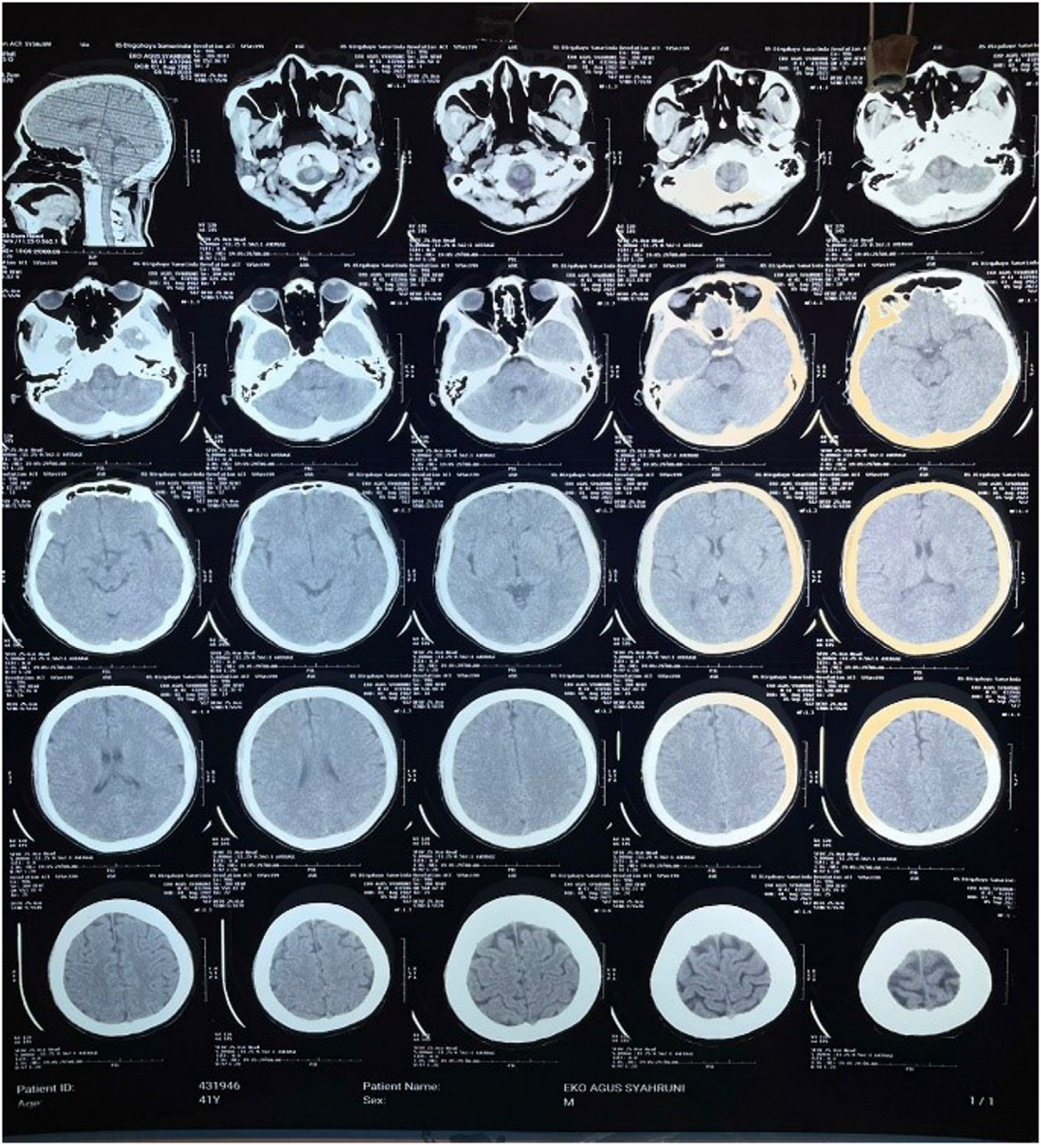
Head computed tomography did not show any focal lesions.
